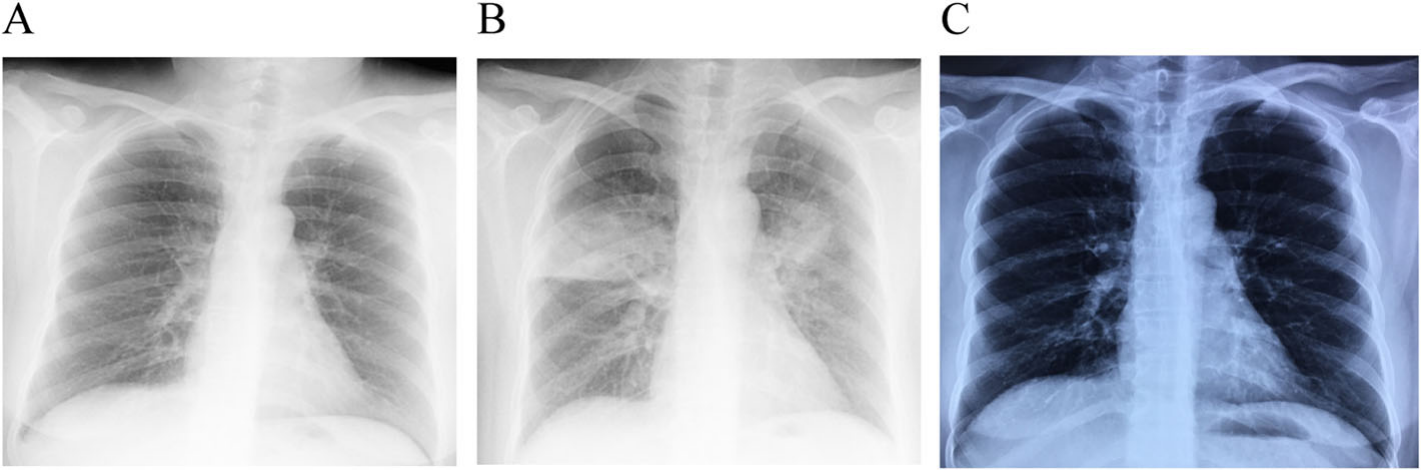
Chest X-ray before or after the final BT session. a There was no remarkable abnormality on chest X-ray before the third session of BT. b Chest X-ray revealed infiltrates newly developed in both upper lobes after both upper lobes BT procedure. c Chest X-ray showed the infiltrates in both upper lobes had diminished after 10-day of antibacterial treatment
